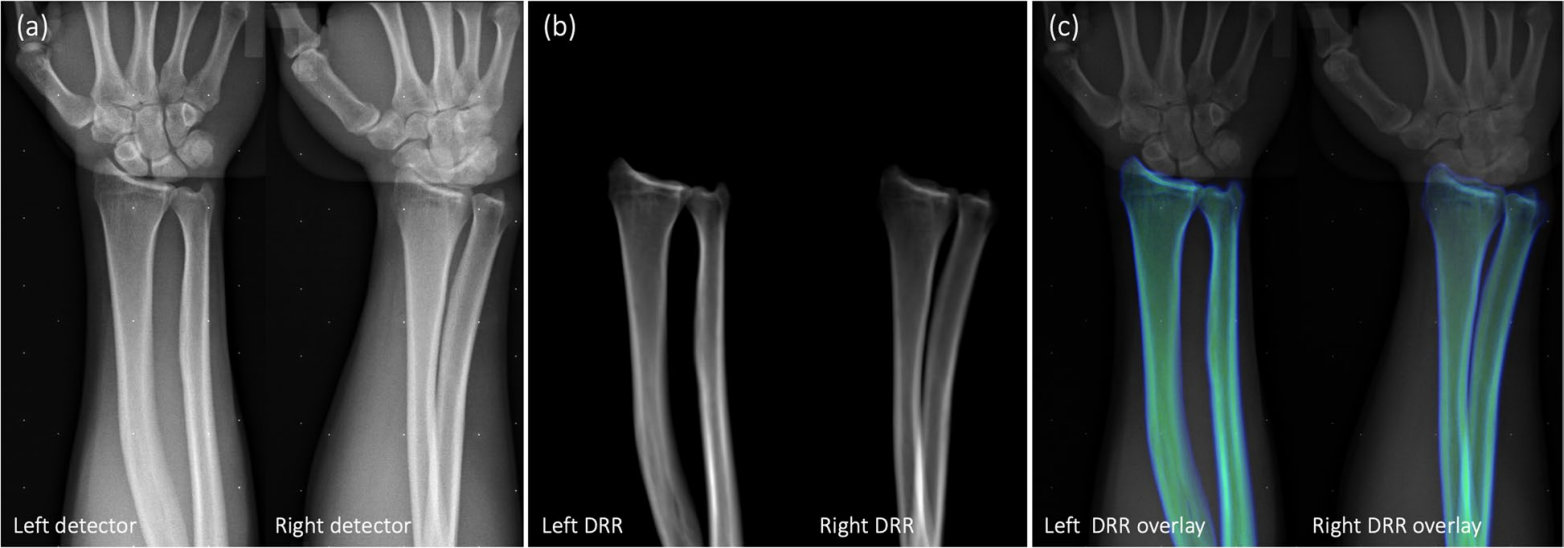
AutoRSA analysis of radiostereometric images. Comparison of (a) radiostereometric images and (b) CT based digital reconstructed radiographs (DRR), was performed with a mathematical algorithm in the AutoRSA software until no further improvements could be made. The optimal overlay (c) was calculated by the AutoRSA software
